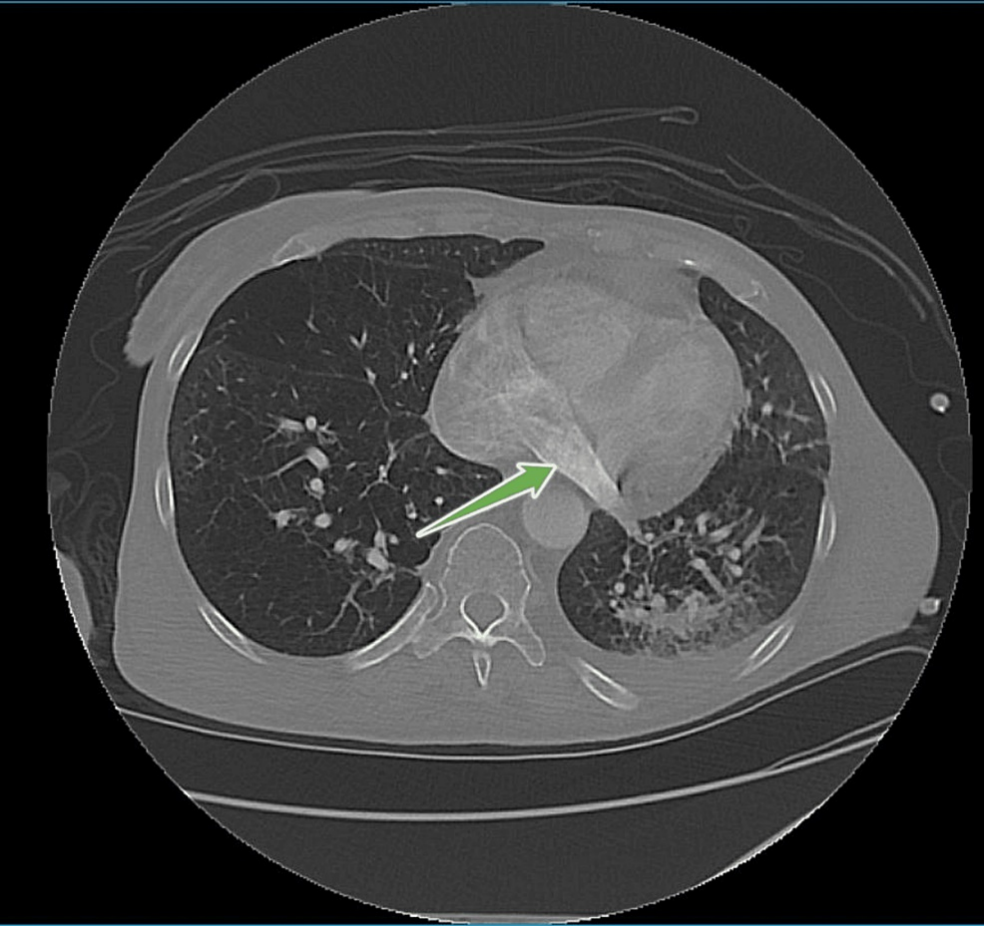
Computed tomography angiography chest demonstrating contrast passing via the left SVC into the coronary sinus and then to the right atrium (green arrow) SVC - superior vena cava
